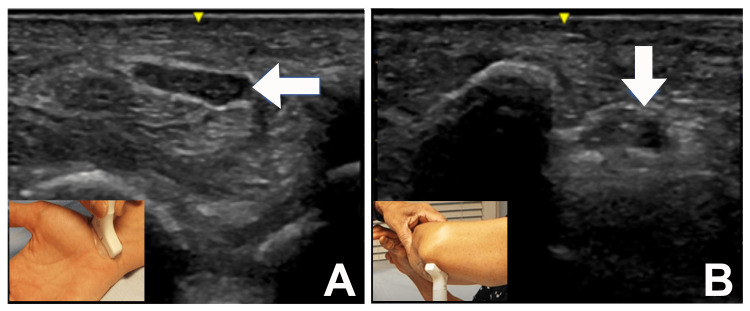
Ultrasound of the median nerve at the wrist and mid forearm Patient 1: Ultrasound of the median nerve at the wrist and mid forearm. (A) Short-axis view of the right median nerve at the carpal tunnel inlet (arrow). The cross-sectional area was 10 mm^2^ (normal: ≤12 mm^2^). (B) Short-axis view of the right ulnar nerve (arrow) at the elbow. The cross-sectional area was 9 mm^2^ (normal: ≤10 mm^2^).
